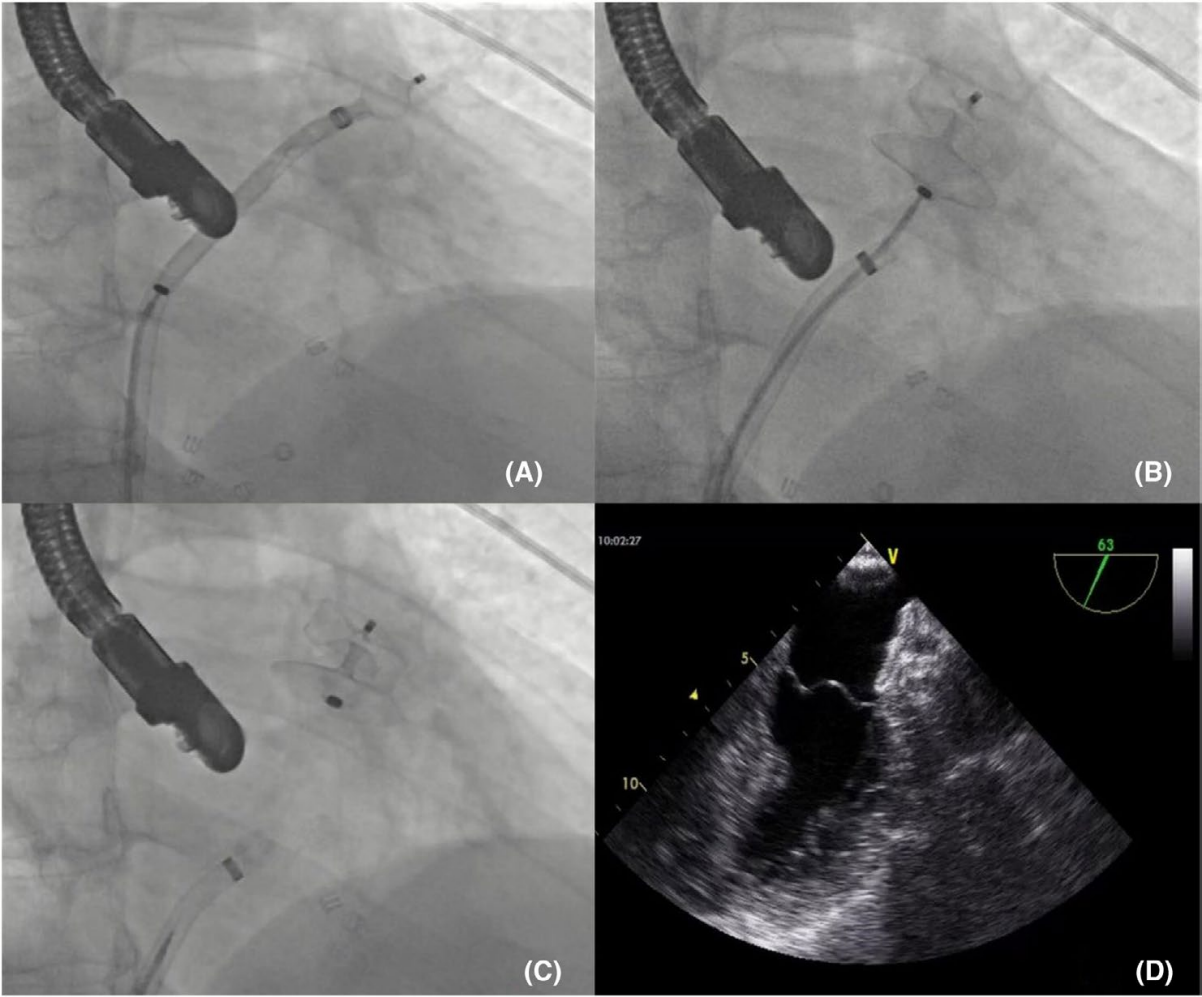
Left Atrial Appendage Closure (LAAC) with the Amulet™ Device in Case 1. (A) The delivery sheath demonstrates the ball‐shaped Amulet™ device. (B) Tug test confirming the stability of the device before release. (C) Final release of the device. (D) Intraoperative transesophageal echocardiography (TEE) image postdevice deployment showing proper positioning and sealing.
